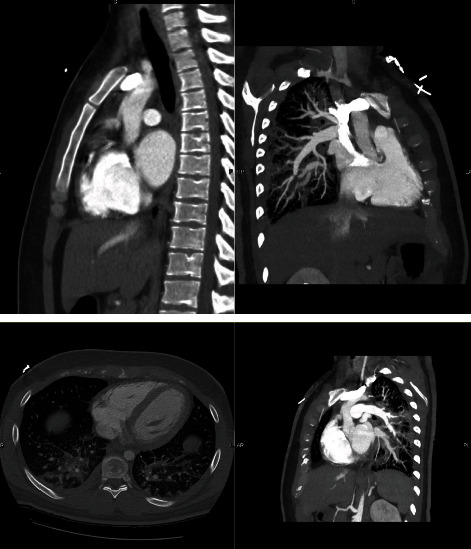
CT Angiography showed perihilar pulmonary infiltrates with ground-glass opacities, mediastinal adenopathy, and splenomegaly.
